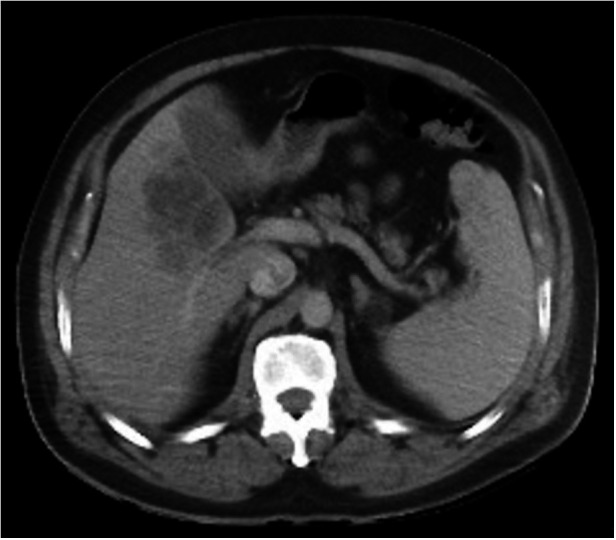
Abdominal computed tomography scan with intravenous contrast, portal phase, showing a hypodense and septated lesion in the hepatic segment V with peripheral enhancement.
